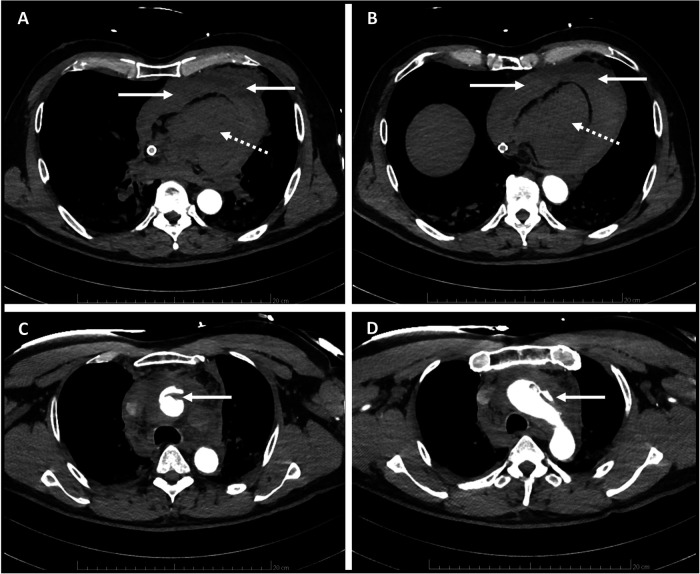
Contrast-enhanced CT scan showing (A,B)
